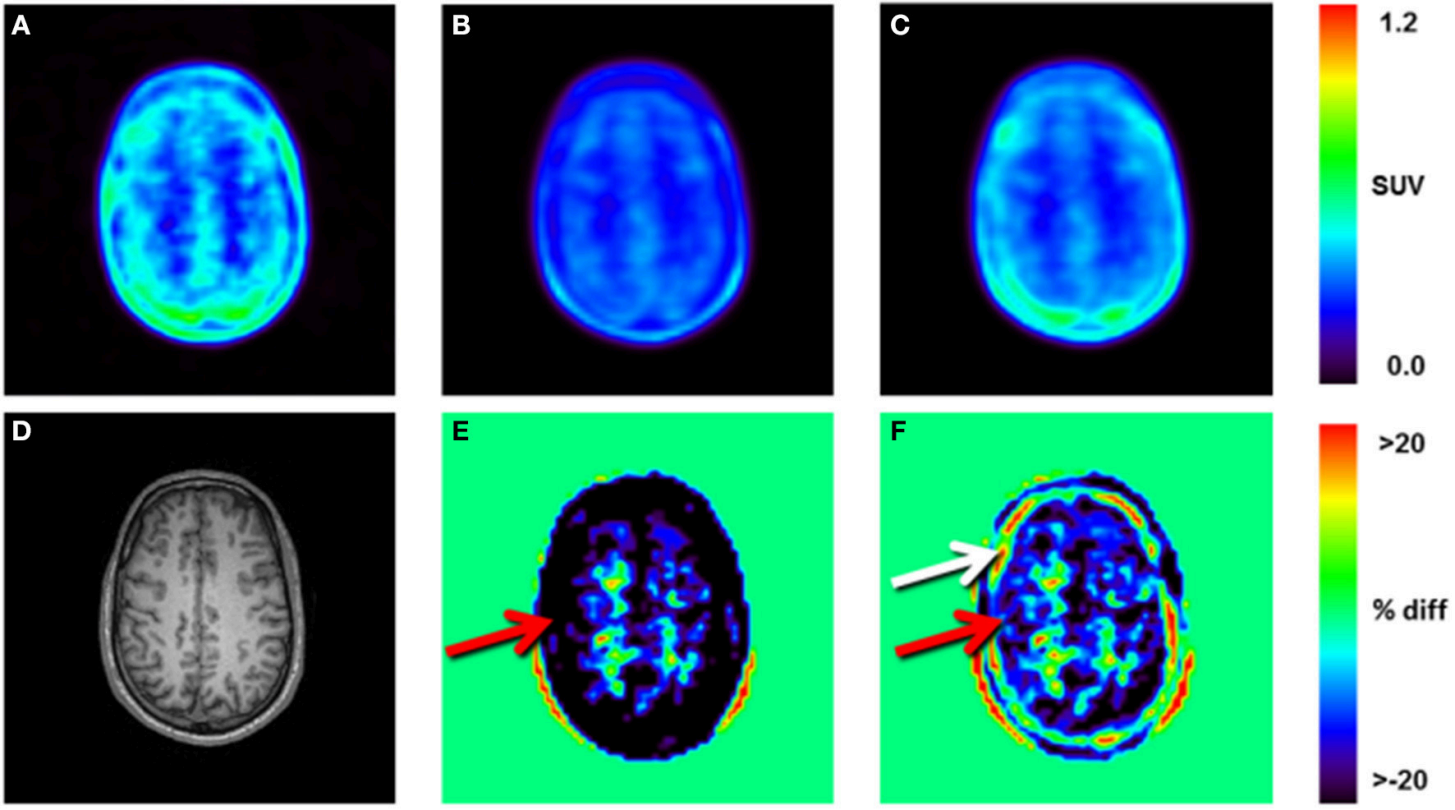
Static reconstructions (10–40 min post injection) of subject 1: (A), PET-only emission data reconstructed using the Tx-ATN map, (B) PET/MR emission data reconstructed using the DIXON-ATN map, (C) PET/MR emission data reconstructed using the CT-ATN map. (D) T1-weighted MR image used for automatic segmentation of the brain-structures, (E) relative differences (%) between B and A, (F) relative differences (%) between C and A. Local differences of up to 55% were observed for areas close to bone, when comparing DIXON- and Tx-reconstructions (red arrow, E). Local differences of more than 25% were observed within the bone between CT- and Tx-reconstructions, indicating differences in the assigned bone-ATN values for the two methods (white arrow, F).
